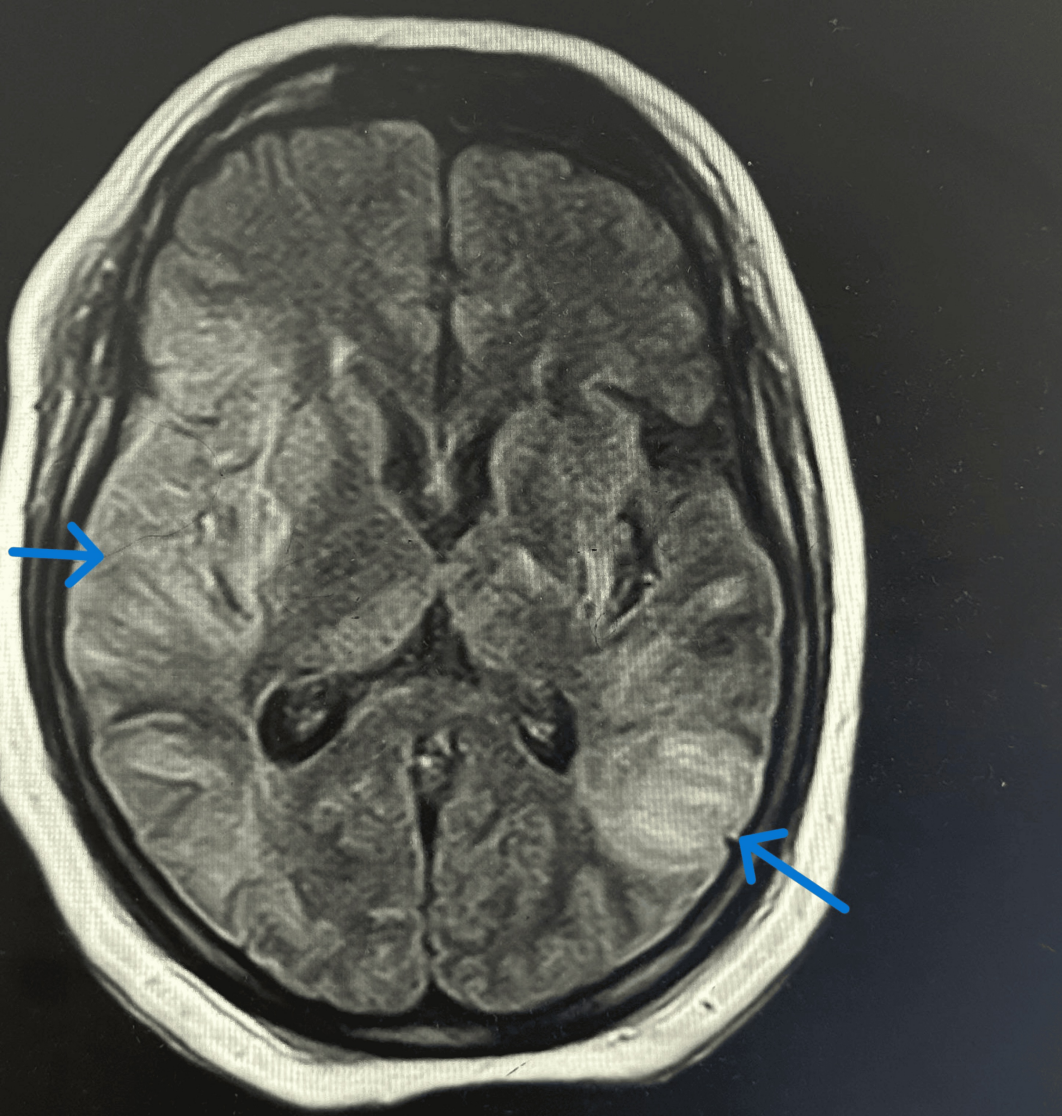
MRI of the brain showing ischemic changes in both cerebral hemispheres.
